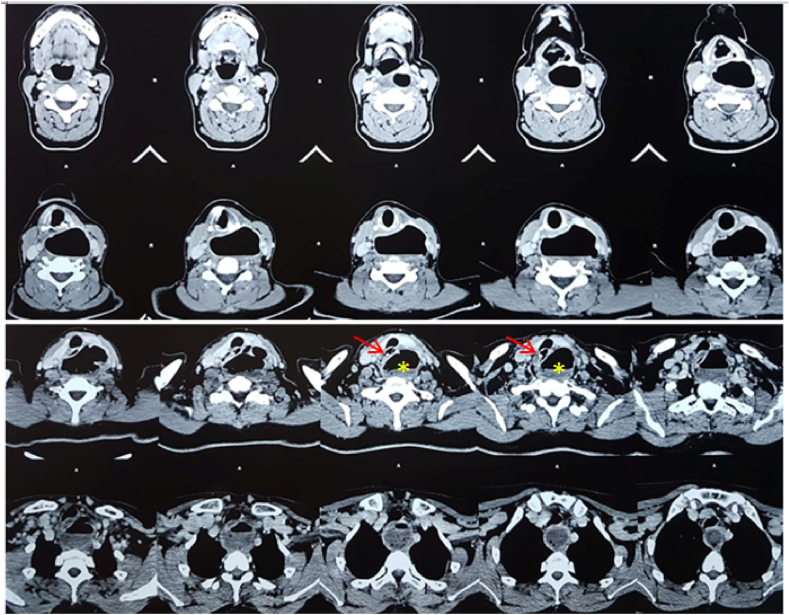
Axial CT scan sections showing the posterior collection with hydroaeric level (yellow *), its extension in the superior mediastinum (orange frame) and the foreign body appearing in calcific density enclosed in the posterior esophageal wall (red arrow).
